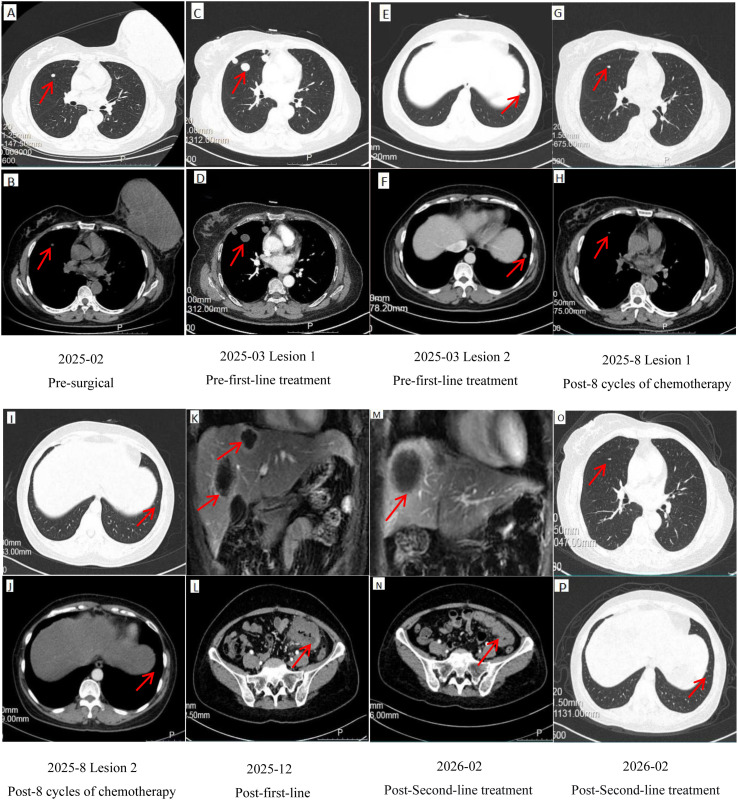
(A, B) CT images of the lung window (A) and the mediastinal window (B) on February 7, 2025; (C, D) CT images of the lung window (C) and the mediastinal window (D) for Lesion 1 pre-first-line treatment on March 18,2025; (E, F) CT images of the lung window (E) and the mediastinal window (F) for Lesion 2 pre-first-line treatment on March 18,2025. (G, H) CT images of the lung window (G) and the mediastinal window (H) for Lesion 1 post-8 cycles of chemotherapy on August 13,2025. (I, J) CT images of the lung window (I) and the mediastinal window (J) for Lesion 2 post-8 cycles of chemotherapy on August 13,2025. (K, L) MRI coronal images (K) of hepatic metastases after first-line progression on December 06, 2025 and CT images (L) of intestinal metastases on December 17, 2025. (M, N) Liquefactive necrosis of the liver on MRI (M) and reduction of intestinal metastatic lesions on CT (N) after two cycles of second-line therapy on February 3, 2026. (O, P) Lung lesions1 (O) and lung lesions 2 (P) after two cycles of second-line therapy on February 3, 2026.
